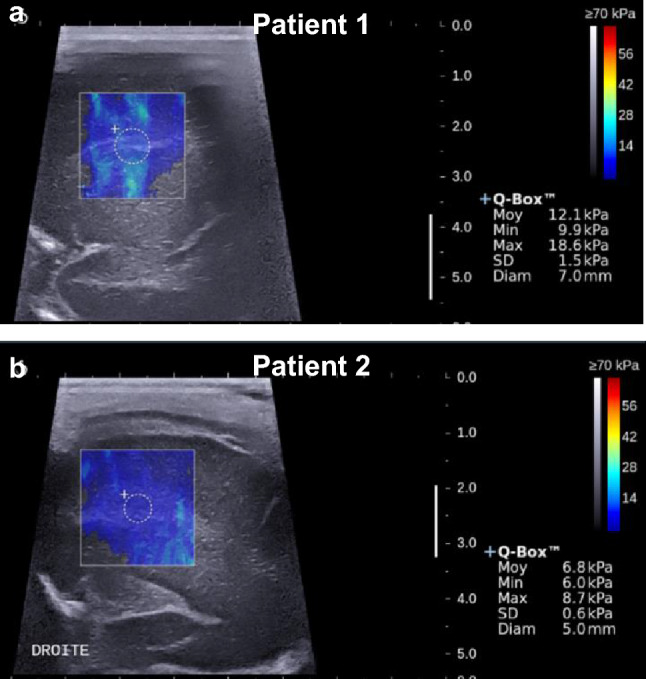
B-mode cranial ultrasound (cUS) images with overlaid shear wave elastography (SWE) measurements at DOL 3 in the right frontal white matter for two patients. B-mode cUS images are shown in grayscale and with overlaid SWE measurements in color-coded map for (a) Patient 1 with normal echogenicity on B-mode cUS image and (b) Patient 2 with abnormal echogenicity on the B-mode cUS image. The information in the Q-box is the summary of the SWE measurements. The corresponding mean elasticity values show that the elasticity is lower in the frontal white matter of Patient 2 with detected abnormal echogenicity (6.8 ± 2.7 kPa) compared to Patient 1 with normal echogenicity (12.1 ± 1.5 kPa).
